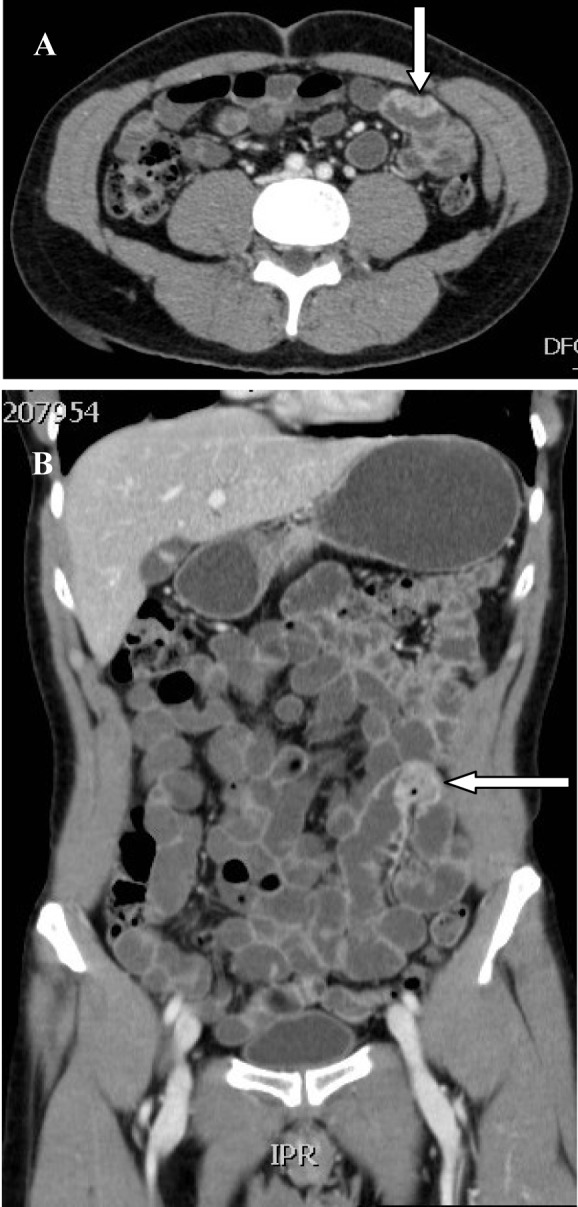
CT-scan showing metastatic tumour to the small intestines resembeling malignant melanoma in axial (A) and coronal sections (B).
